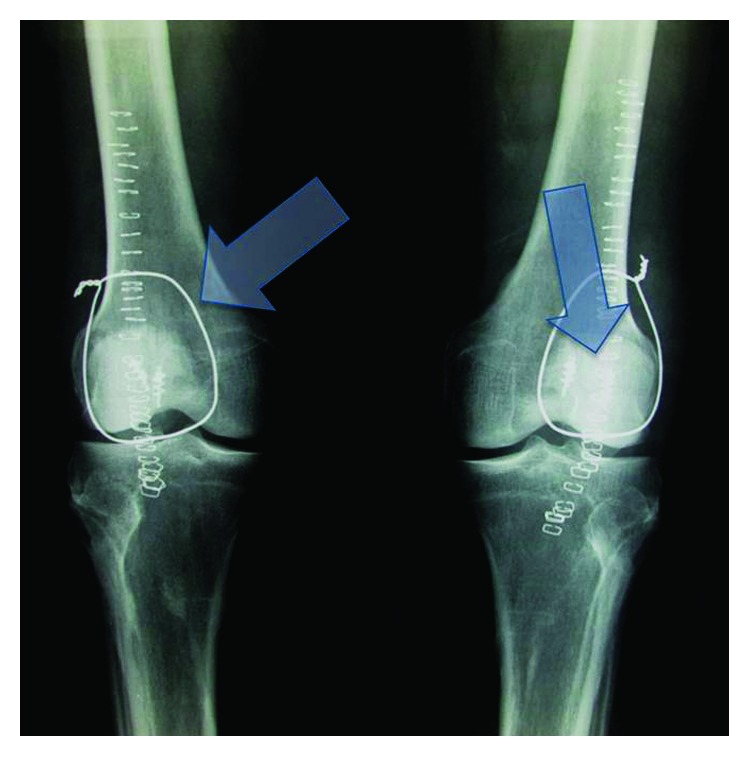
Early postoperative X-rays of the knees. Left arrow shows a cerclage wire, and right arrow shows the two 3.5 mm suture anchors.
